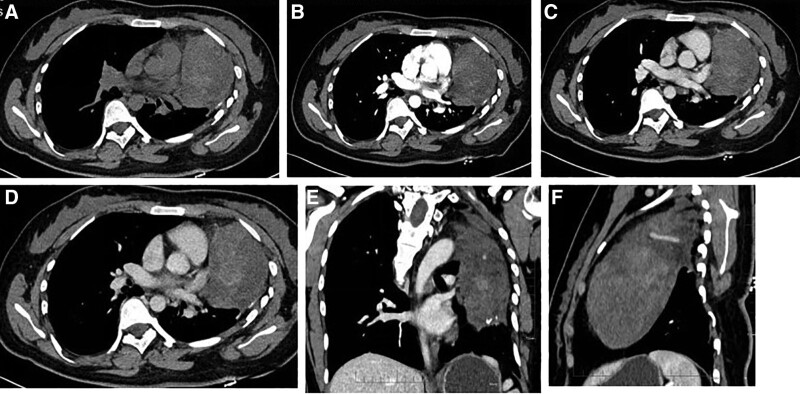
CT showed a mass of mixed density in the left thoracic cavity with calcification and fat density, and the boundary with the mediastinum was not clear. The enhancement was uneven, and the thick blood vessels were seen in the left thoracic cavity. CT = computed tomography.
